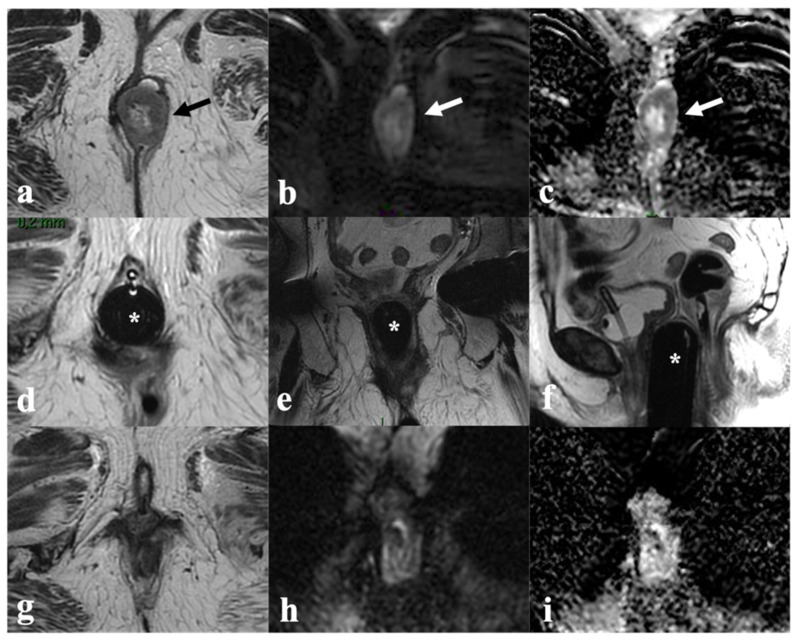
Complete response to CRT and brachytherapy in recurrent vulvar cancer. An 80-year-old patient with recurrent vulvar cancer after radical vulvectomy and lymphadenectomy, presenting with intermediate T2 solid tissue in left vulvar region ((a)—black arrow) with restricted diffusion in DWI and ADC map ((b,c)—white arrow). After CRT, brachytherapy was performed, with MRI monitoring after applicator insertion ((d–f)—white star key); axial (d) coronal (e) and sagittal (f) T2 images show that the applicator is correctly positioned, and no residual disease is visible. MRI at treatment completion shows tumor disappearance in both T2WI (g) and DWI/ADC map (h,i).
